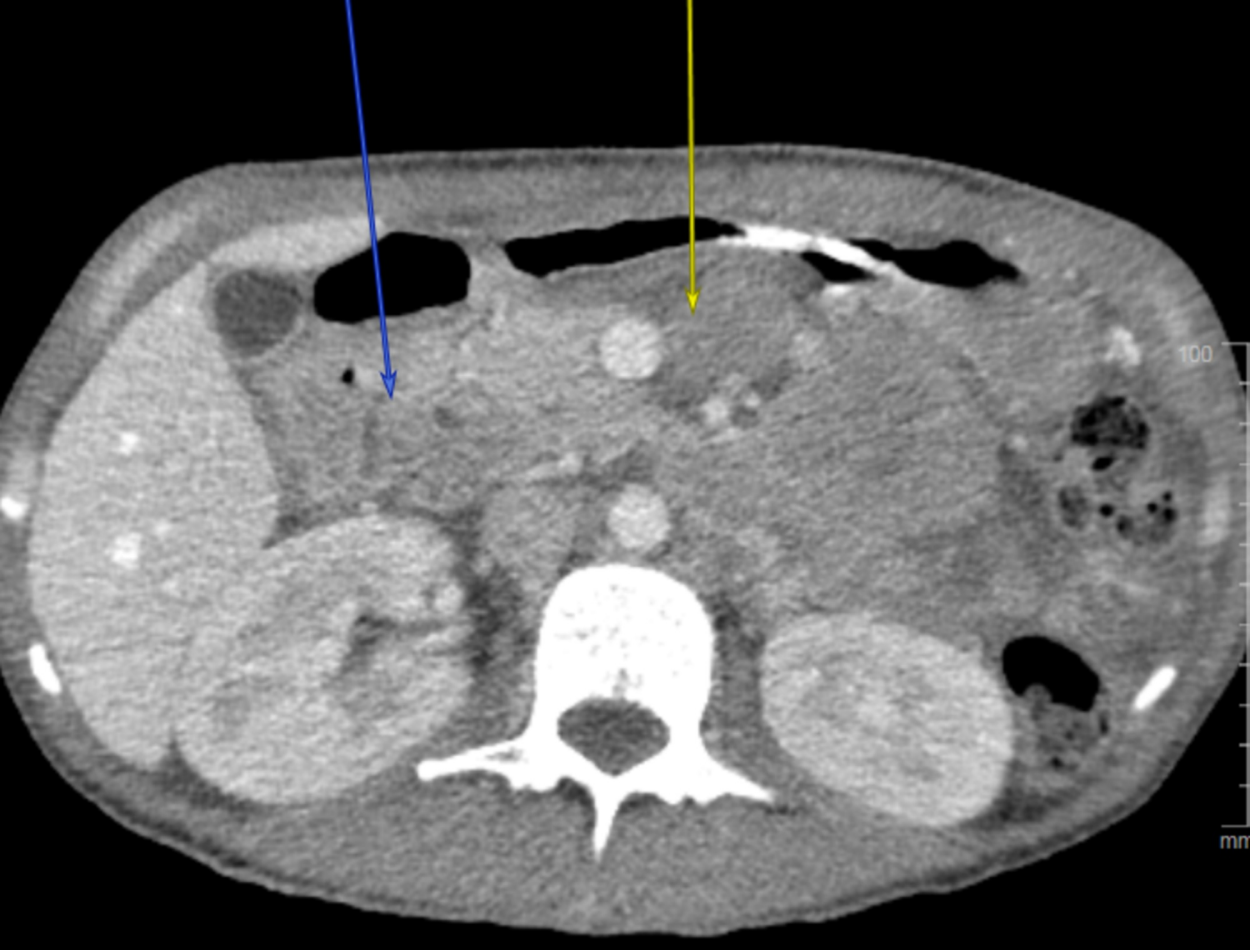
Peritoneal lymphomatosis Computed tomography image showing multifocal masses throughout the mesentery and centered within the peritoneum, compatible with “carcinomatosis.”
